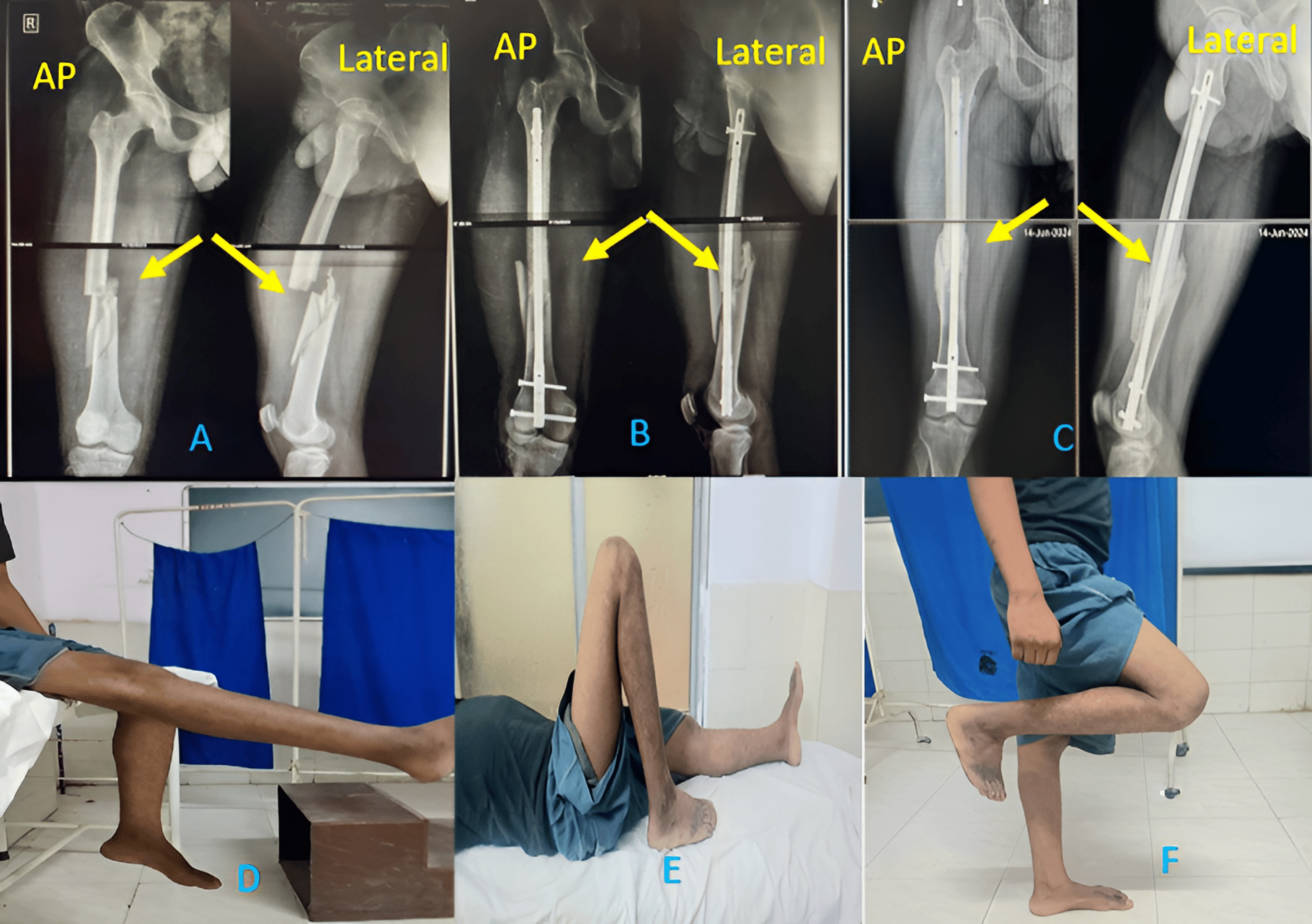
Excellent results obtained with IMSCN (A) AP and lateral X-rays after trauma (arrow showing fracture), (B) AP and lateral X-rays after the surgery (arrow showing the fracture), (C) AP and lateral X-rays at the final follow-up (arrow showing union at the fracture site), (D) Knee extension at the final follow-up, (E) Knee flexion at the final follow-up, and (F) Knee flexion in standing position IMSCN: Intramedullary Supracondylar Nail
